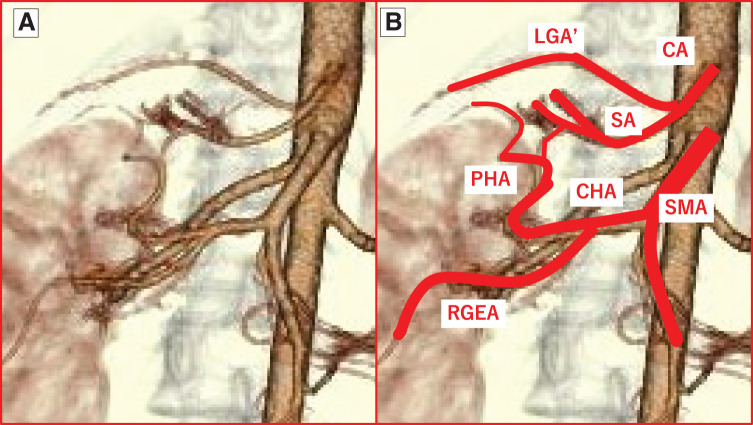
3D CT angiography of abdominal vasculature. (A) Front view after discharge. (B) The CA and SMA are highlighted with red lines. After coursing toward the right posterior side, the CA divides into an upper branch—presumed to be an LGA’—and a lower branch, the SA. The SMA divides into right and left branches. The right-hand branch represents the CHA, which further divides into upper and lower branches. The upper branch curves in an arc toward the head and continues as the PHA, while the lower branch passes caudally through the pancreatic head and becomes the RGEA. CA, celiac artery; CHA, common hepatic artery; LGA, left gastric artery; LGA’, variant left gastric artery; PHA, proper hepatic artery; RGEA, right gastroepiploic artery; SA, splenic artery; SMA, superior mesenteric artery
